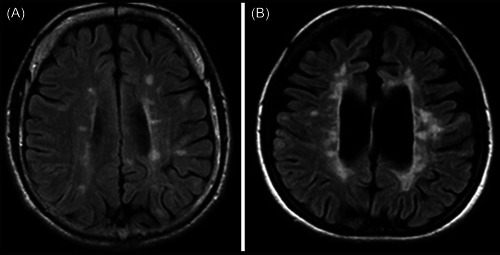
Brain MRI results in primary‐progressive multiple sclerosis (PPMS) patients are equivalent to those in relapsing‐remitting multiple sclerosis (RRMS) and secondary progressive multiple sclerosis (SPMS) patients. Brain MRI images of PPMS patients with varied FLAIR pictures, and axial plane MRI presentations. (A) The deep, juxtacortical, and periventricular white matter all had many isolated lesions. This MRI is equivalent to the standard MRI scan performed on RRMS patients; (B) An axial plane image of a FLAIR. The periventricular white matter included localized and confluent lesions, and some of the lesions in the juxtacortical regions resembled those seen in SPMS. There are indicators of brain atrophy as well, Figure from Siger.
